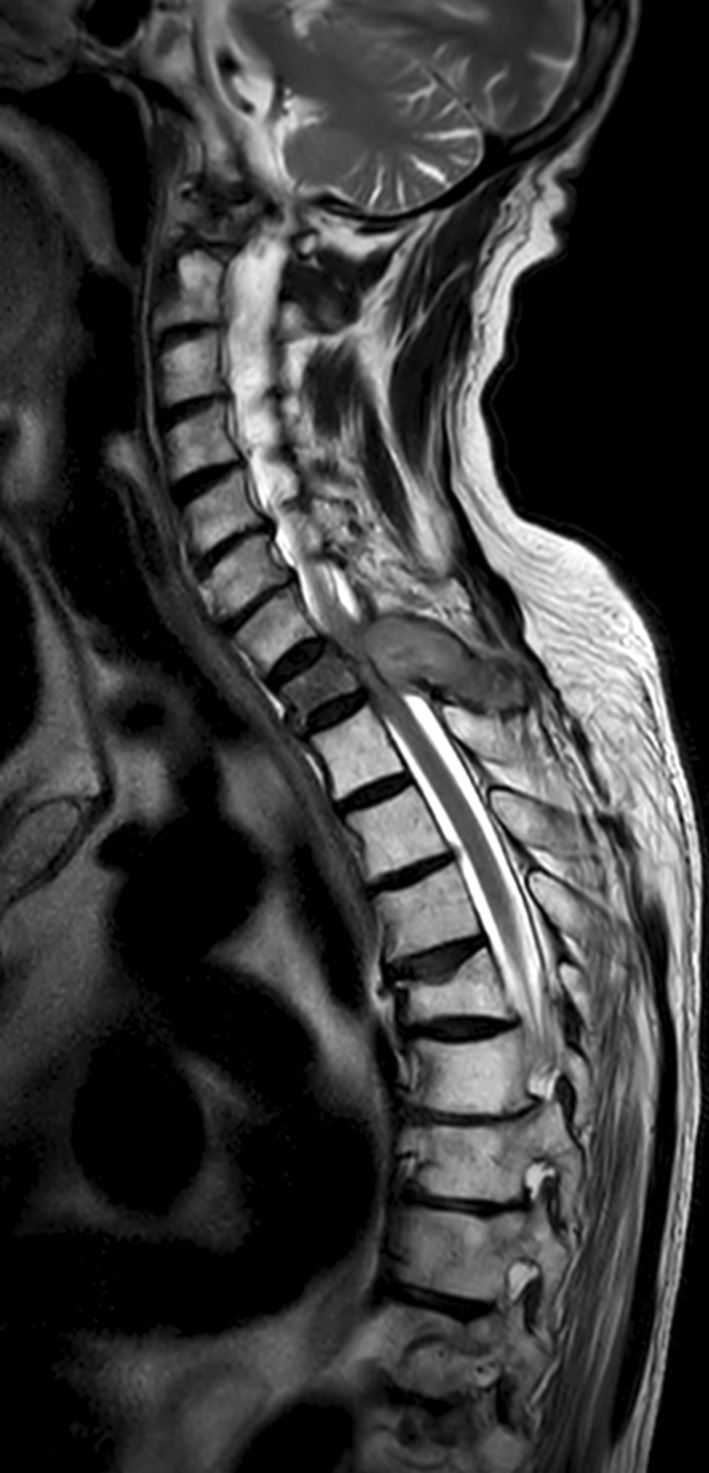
T2‐weighted MRI showing acute compromise of the spinal cord at the thoracic level, with pathological fracture of the T1 vertebra. A subacute fracture of T5 is also apparent.
